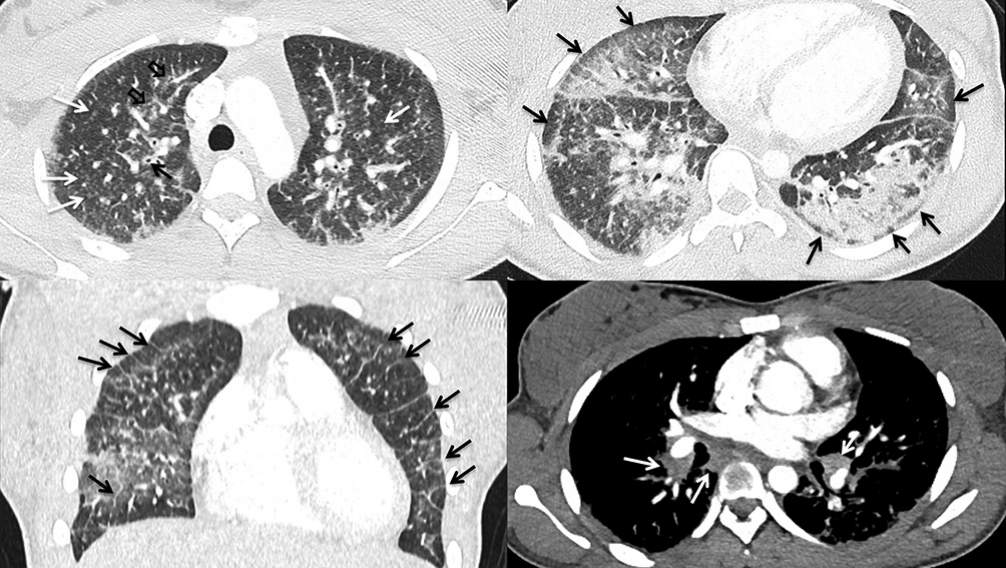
A (top left). Axial HRCT image showing both centriolbular (white arrows) and peribronchovascular (black open arrows) and severe bronchial wall thickening in the upper lobes B (top right). Axial HRCT image showing lower lung predominant peribronchovascular groundglas and consolidation with subpleural sparing (black arrows) C (bottom left). Coronal CT image showing bilateral groundglass opacities with septal thickening (black arrows) D (bottom right). Axial CT image showing mild subcarinal and bihilar lymphadenopathy. HRCT, high-resolution CT.
